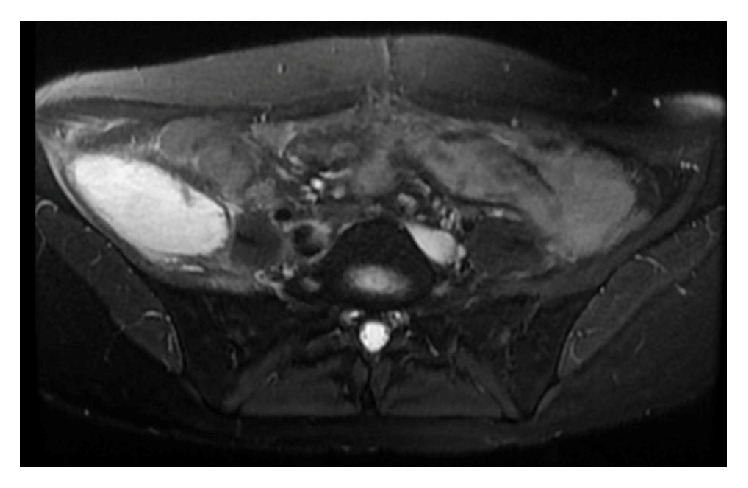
MRI showing axial view of right iliacus muscle sterile seroma.
